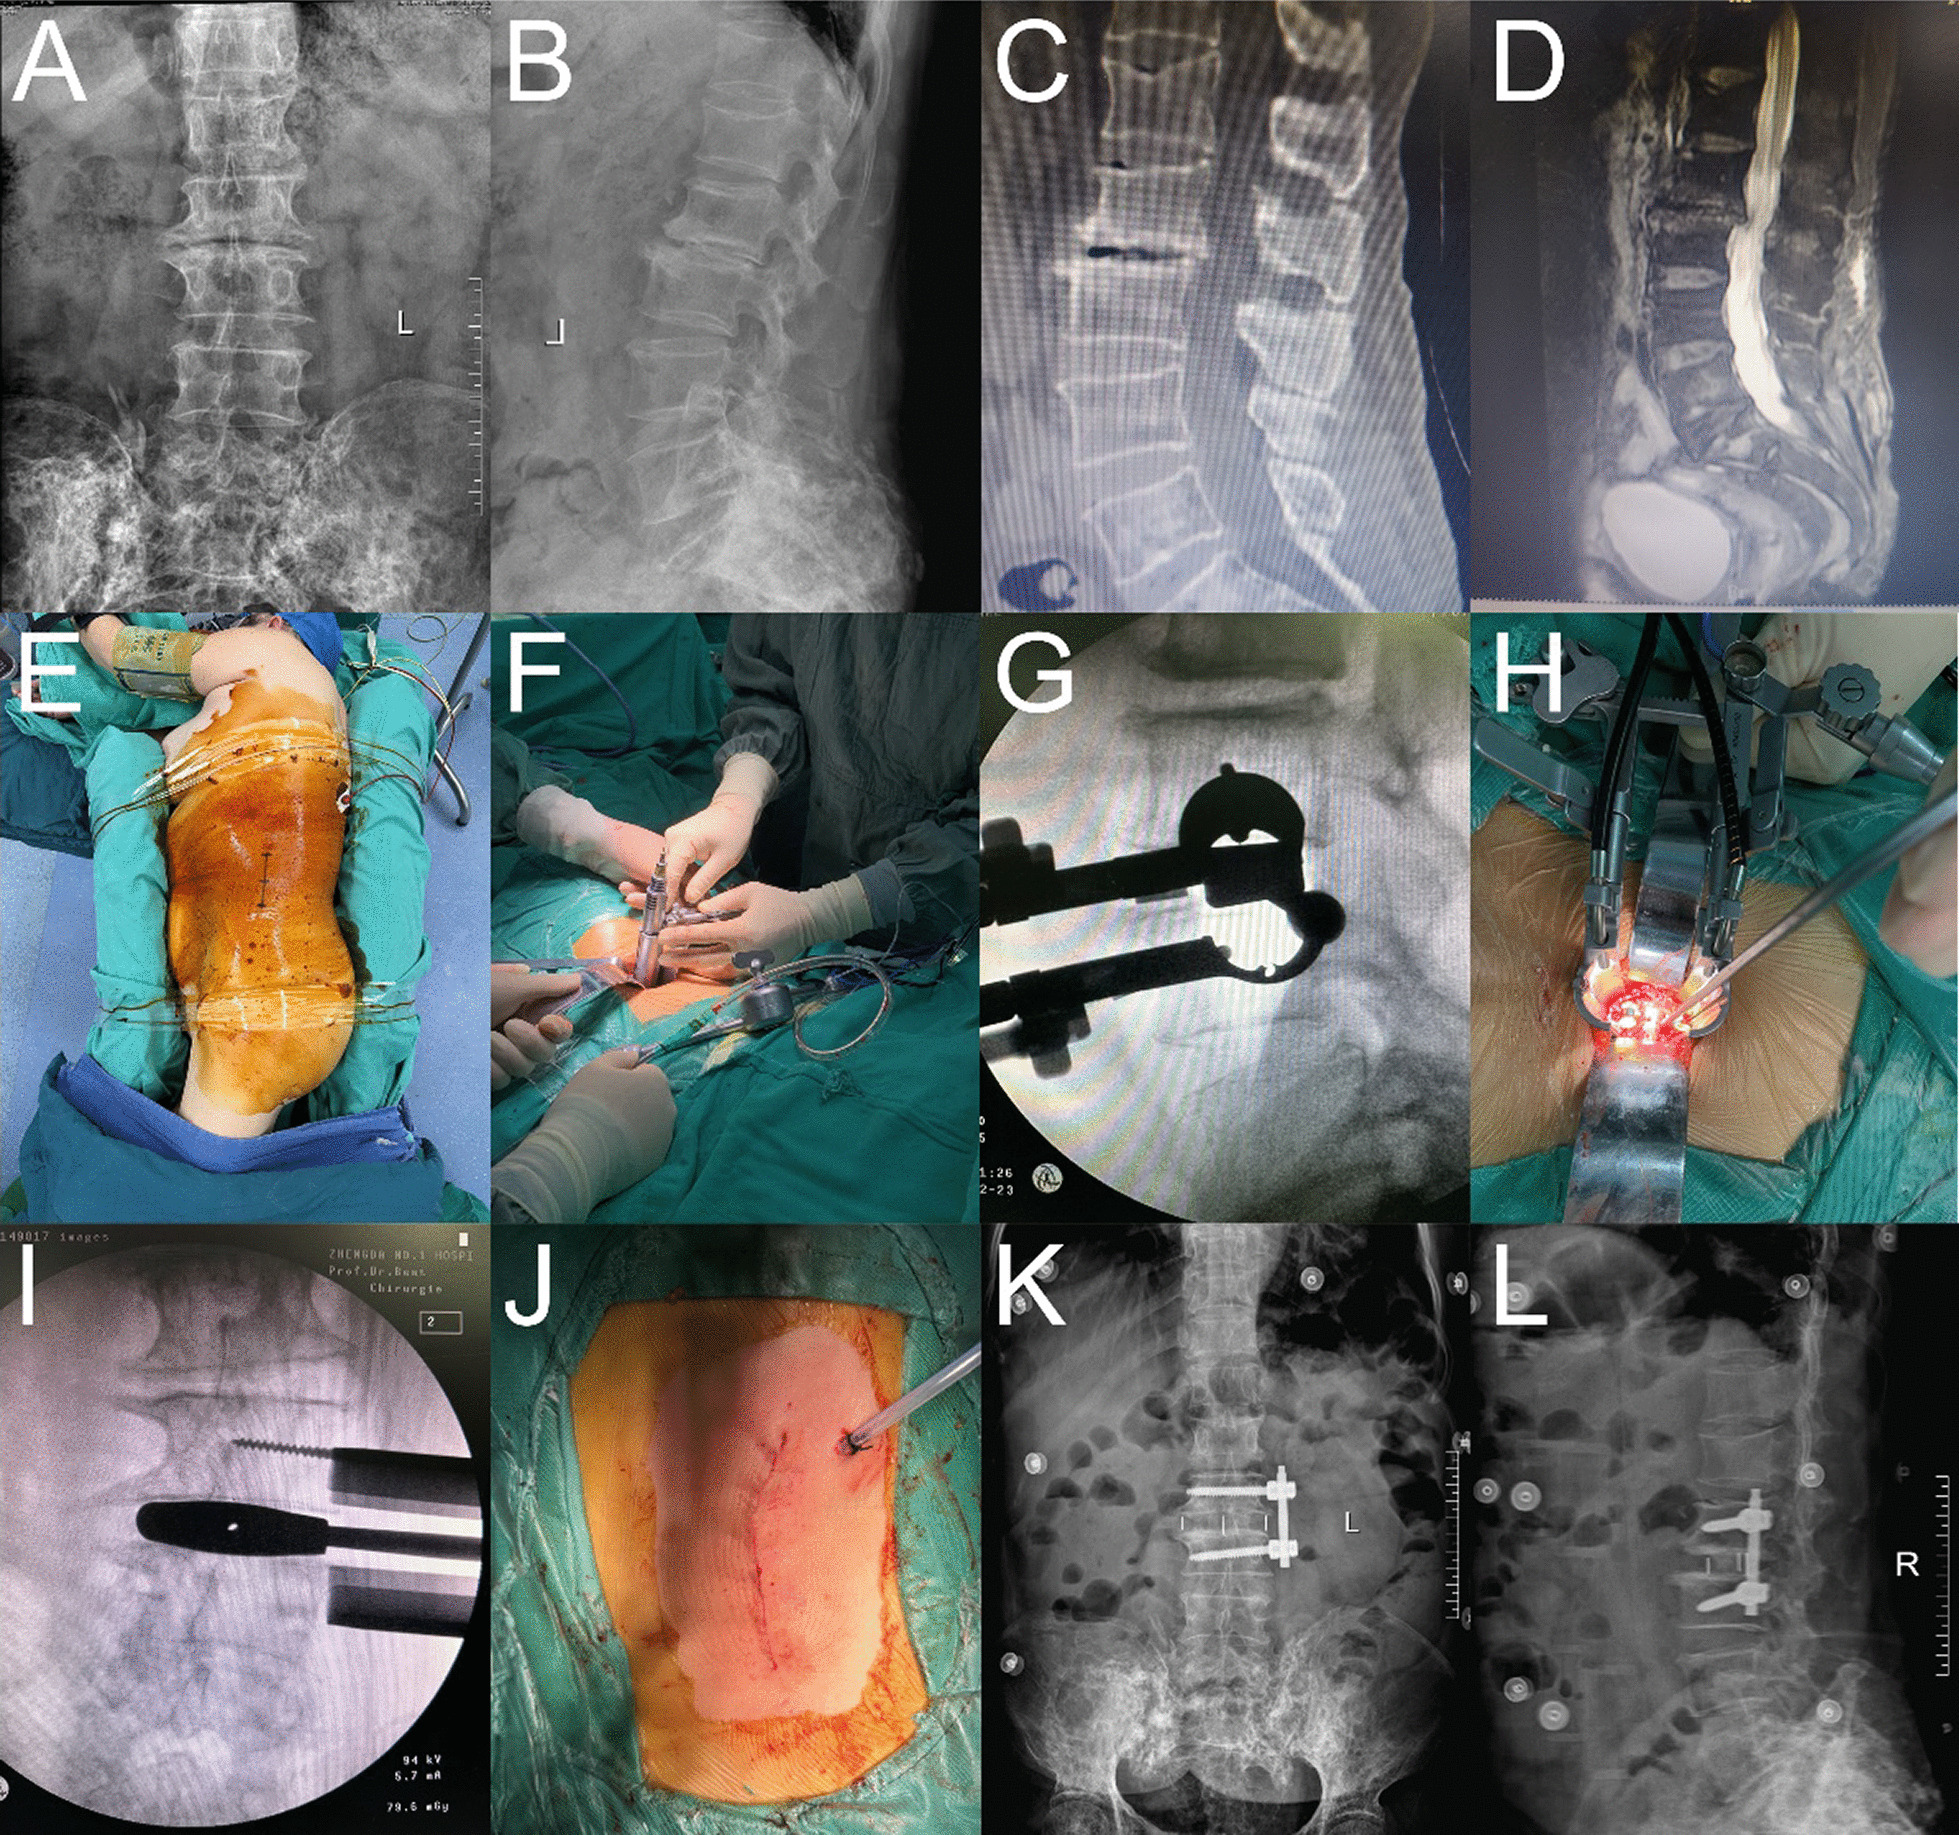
OLIF-AF surgical procedure. A, B: DR in preop; C: CT in preop; D: MRI in preop; E: marked the body surface; F: insert the sleeve; G: confirmed the intervertebral space; H: endplate preparation; I: implanted cages; J: sutured the incision; K, L: DR in postop reexamination
